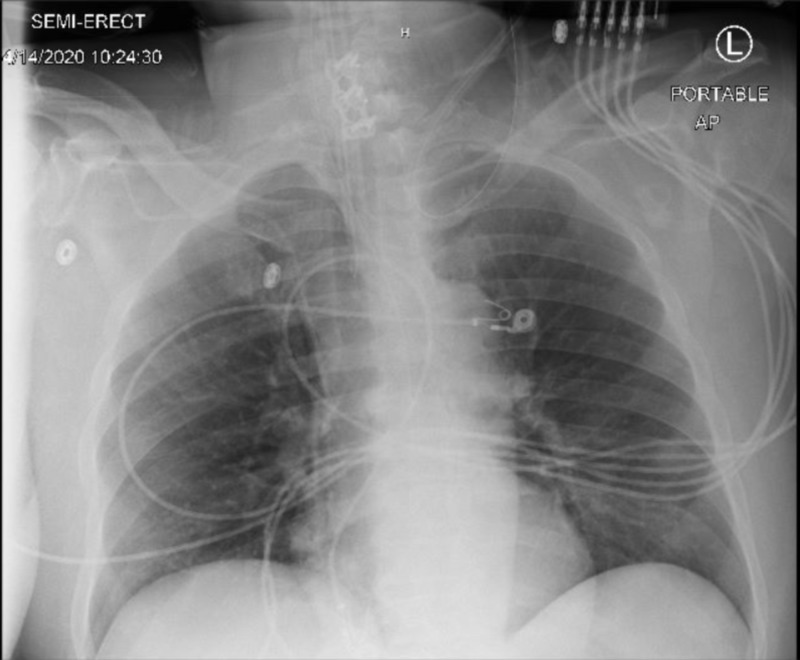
Chest X-ray is unremarkable for infiltrates.
